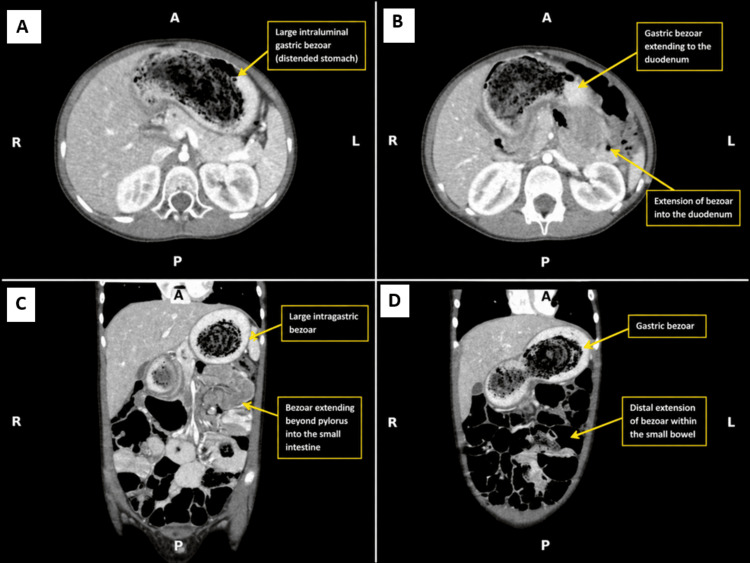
Contrast-enhanced CT of the abdomen demonstrating Rapunzel syndrome with gastric trichobezoar extension into the jejunum, intussusception, and pancreatic compression (A, B) Axial contrast-enhanced CT images demonstrate a large mottled intraluminal gastric trichobezoar occupying the stomach with extension beyond the pylorus. (C, D) Coronal reformatted images demonstrate the elongated bezoar tail extending into the proximal jejunum, consistent with Rapunzel syndrome, with associated small bowel telescoping/intussusception and mass effect on the pancreatic region. Orientation markers: A = anterior, P = posterior, R = right, and L = left.
